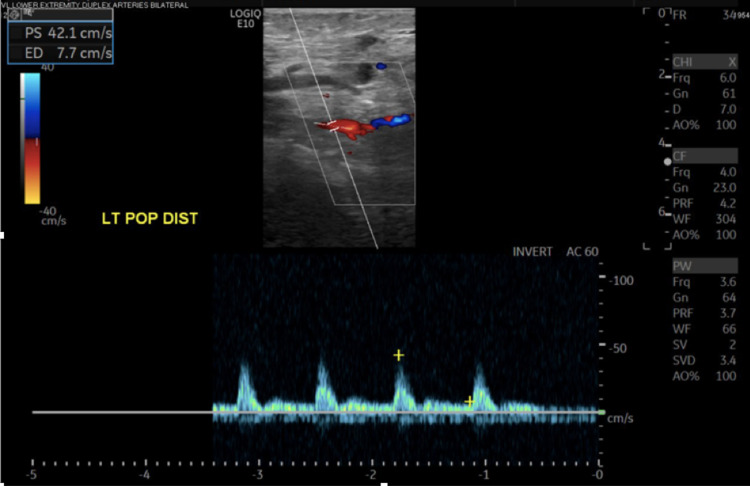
Lower extremity Doppler. Improved blood flow in the left distal popliteal artery after heparin treatment.
